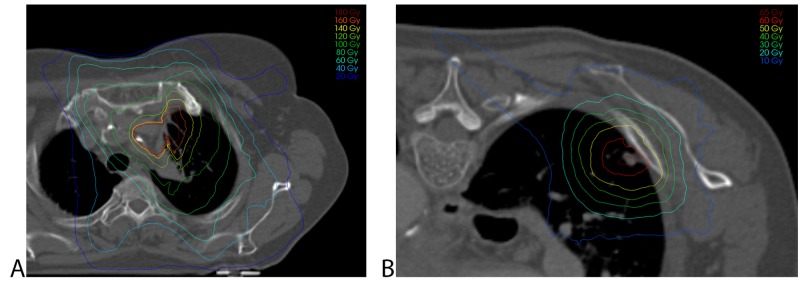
Dosimetric details of the irradiation A) Accumulated dose projected on the planning CT scan of the conventional planning, showing 100 - 140 Gy EQD_2_ (α/β-ratio of 3 Gy) in the sternoclavicular joint; B) Planning CT scan for stereotactic radiotherapy to the right upper lobe in absolute dose. Maximum dose in the fifth rib is 306 Gy EQD_2_ (α/β-ratio of 3 Gy). EQD: equivalent dose
